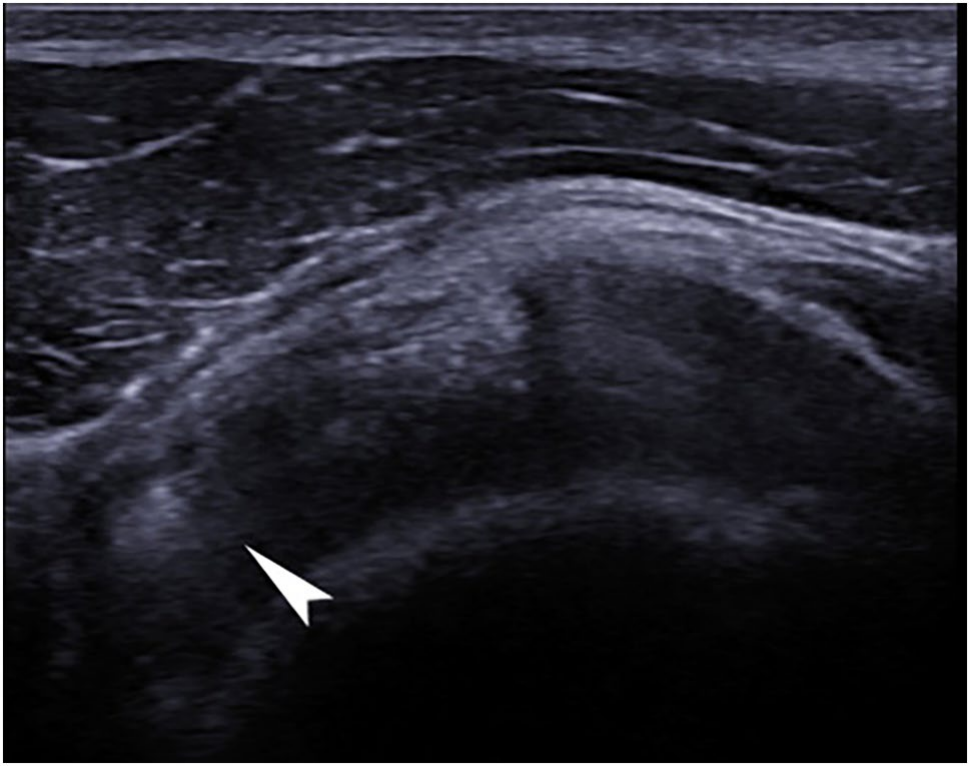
Arm in forced internal rotation, the probe on the coronal plane focusing on the right supraspinatus tendon. Ultrasonography shows hyperechoic formation with posterior enhancement (arrow)—calcification
